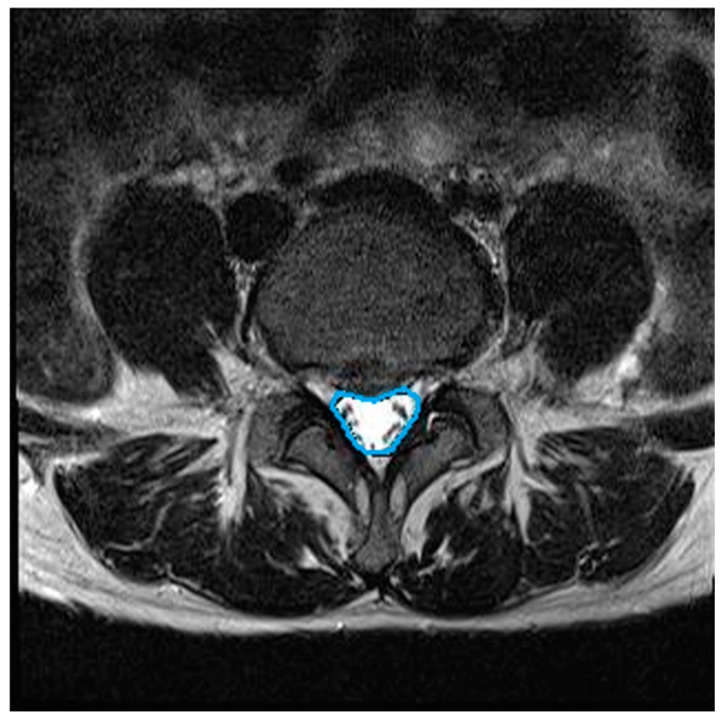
Central canal area in axial view of lumbar spine MRI (Magnetic Resonance Imaging).
